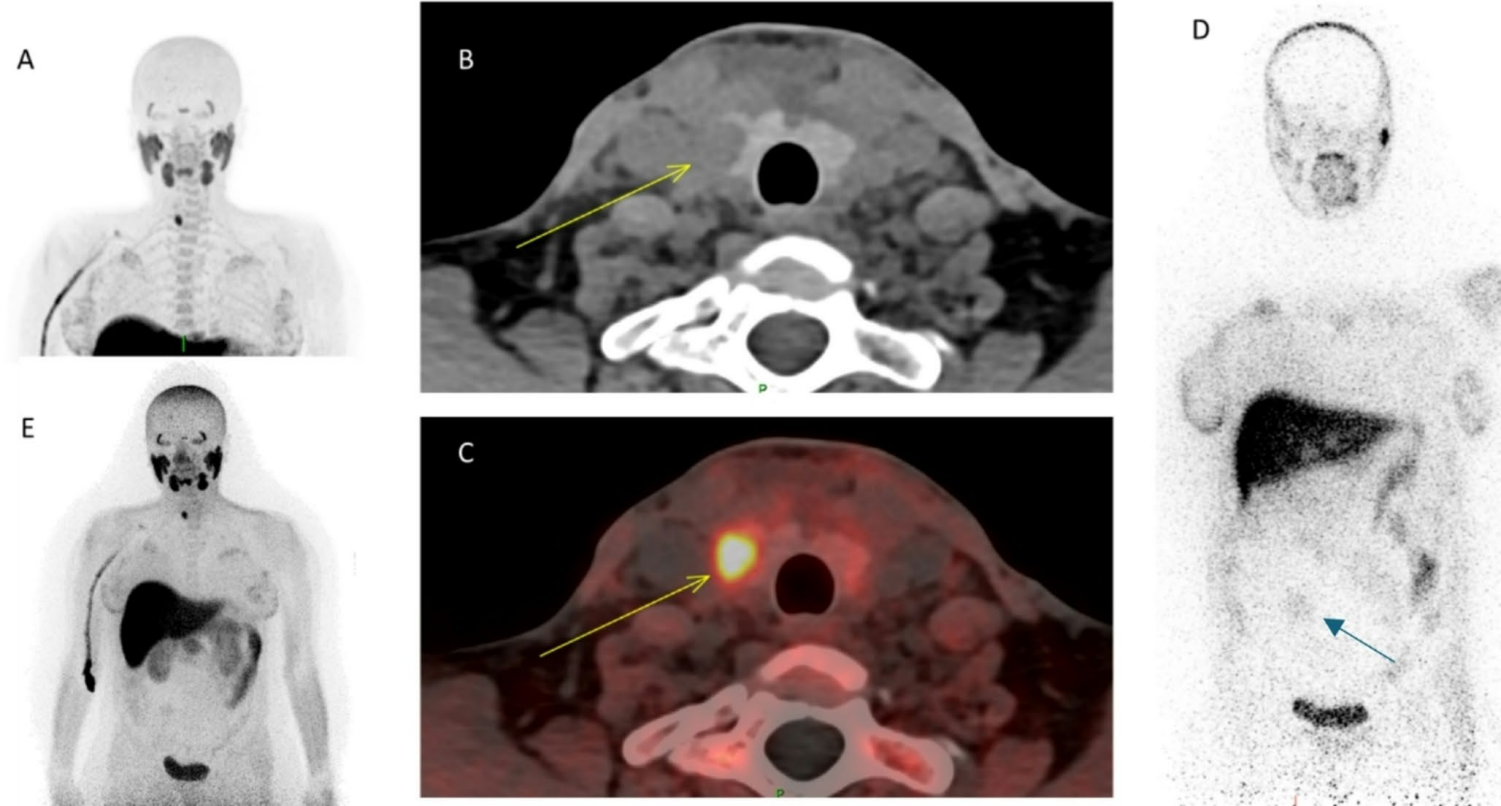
A 44-year old pregnant patient with primary hyperparathyroidism causing severe hypercalcaemia was referred for [^18^F]-fluorocholine long-axial-field of view (LAFOV) PET-CT for pre-surgical localisation of parathyroid adenoma [1]. The patient needed multiple hospitalisations and was at risk of foetal and maternal complications. Exploratory neck surgery was unsuccessful.
